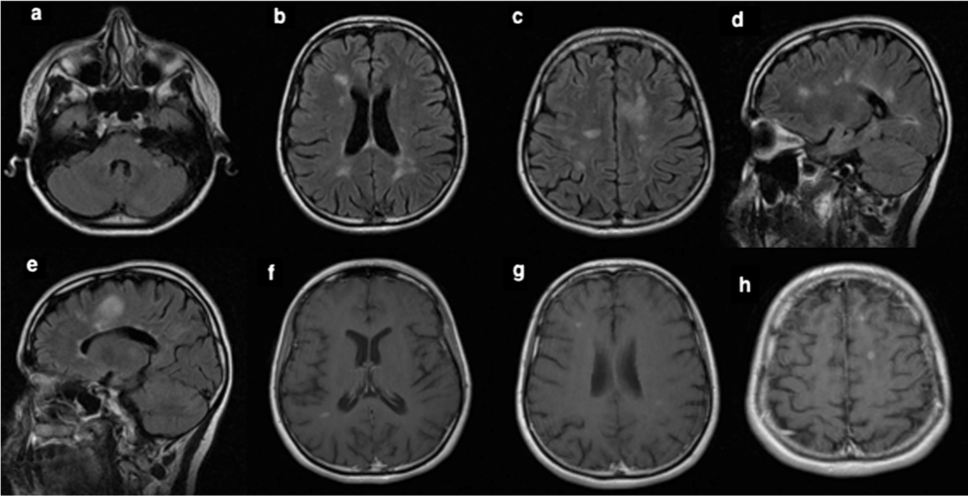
Follow-up MRI brain scans (April 2013) showed new T2/flair lesions in periventricular and juxtacortical regions with an increase in the overall lesion load when compared to the previous MRI scan as seen in the axial (a-c) and sagittal images (d-e). Enhanced Axial T1 MRI images showed five gadolinium-enhancing supratentorial lesions involving both hemispheres (f-h).
